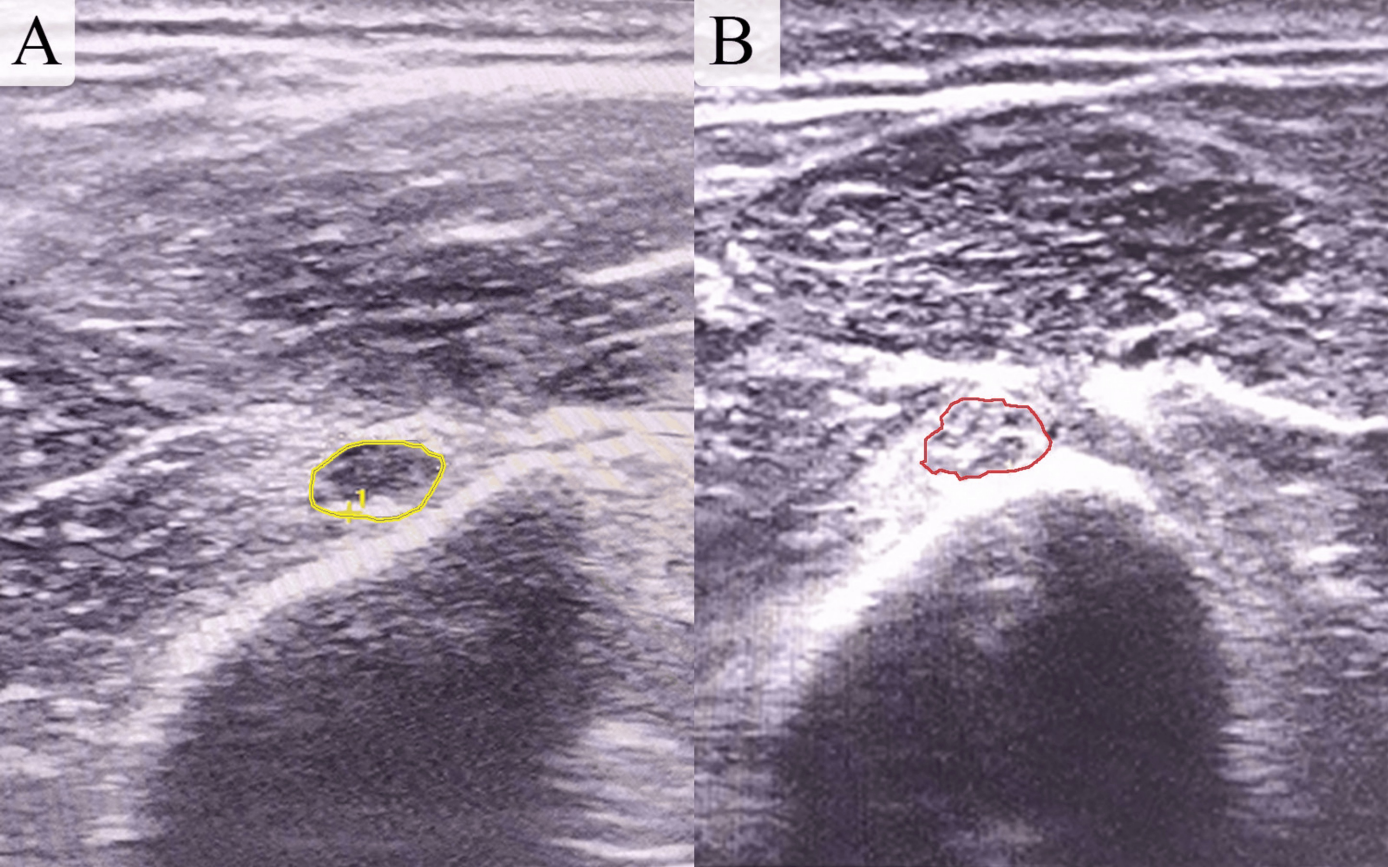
Comparative ultrasound images show a decrease in swelling and a reduction in the cross-sectional area (CSA) of the radial nerve, which decreased from 0.22 cm² at the time of the first injection (image B) to 0.13 cm² two months later (image A).
